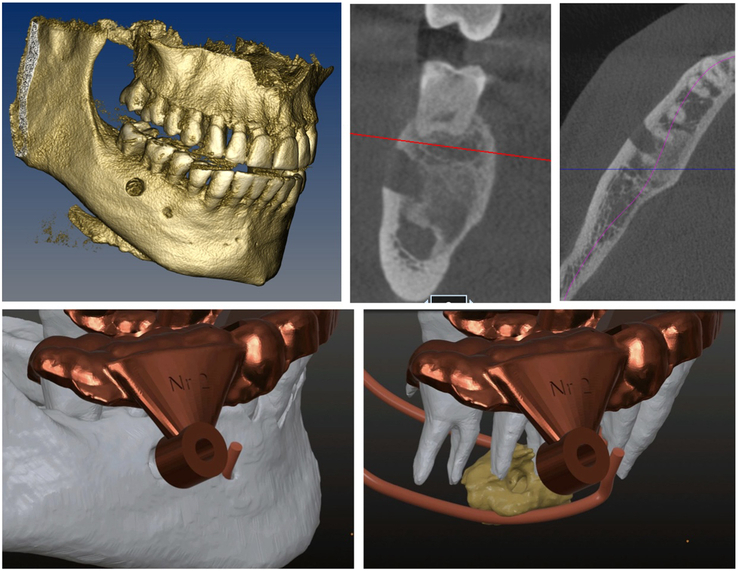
Postoperative CBCT in axial and coronal plane shows the correct removal of the pathologic tissue and the respect of the roots of tooth 4.6 and the mandibular canal. The 3D reconstruction highlights the entrance foramen. The visualization of the 3D reconstruction imported into Nomad Sculpt software demonstrates the consistency between digital planning and surgical execution.
